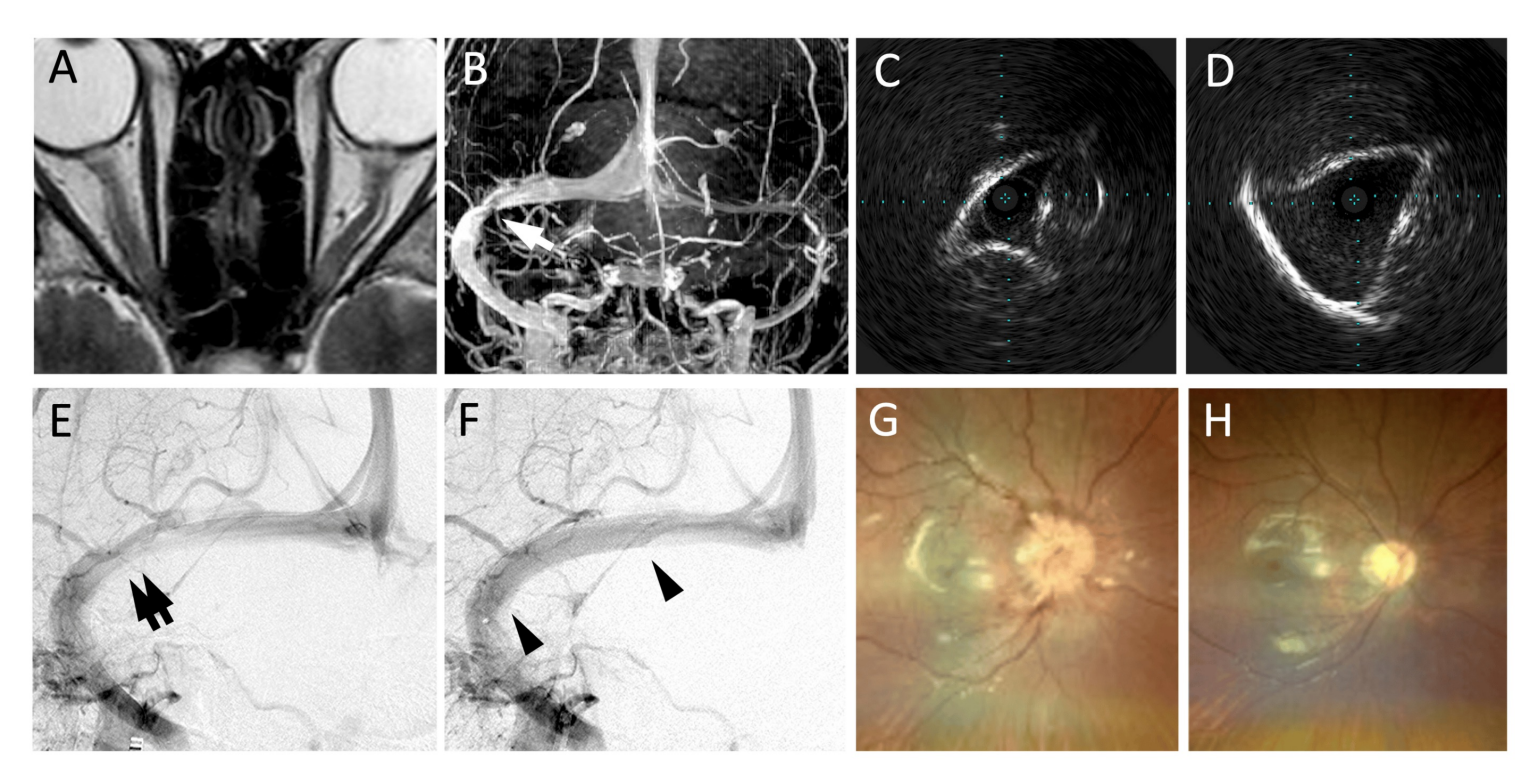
Images from Case 2 (A) MRI on admission displayed optic nerve tortuosity, enlargement of the peripapillary subarachnoid space, and flattening of the dorsal sclera of the eyes. (B) Contrast-enhanced MRI venography demonstrated stenosis of the right transverse sinus (arrow), whereas the left transverse-sigmoid sinus was hypoplastic. (C) IVUS image shows the triangular extrinsic stenosis at the transverse-sigmoid junction. (D) IVUS image provides precise measurements of the medial part of the transverse sinus, helping determine the stent size. (The distance between blue dots represents 1 mm.) (E) Right internal cerebral angiogram demonstrated stenosis of the transverse sinus (double arrow). (F) Angiogram after stenting exhibited the dilatation of the transverse sinus. The stent (between the two arrowheads) did not disturb the flow of the vein of Labbé. (G)-(H) Ophthalmoscopic examination revealed severe papilledema on admission (G) and its recovery at postoperative day 20 (H). MRI: magnetic resonance imaging, IVUS: intravascular ultrasonography.
